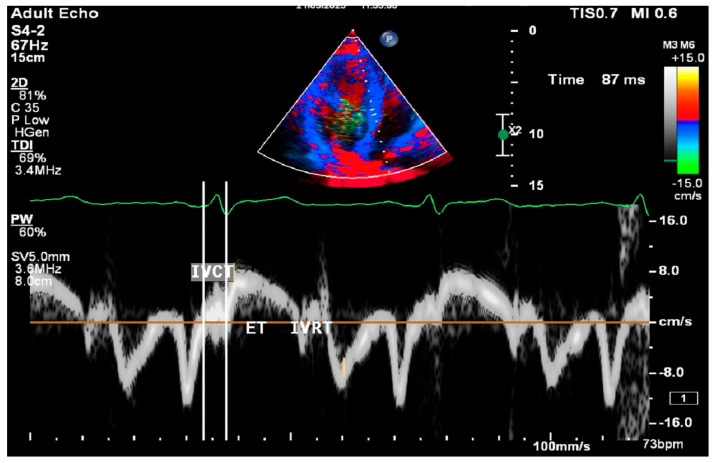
Test image in conventional M-mode, B-mode, and Doppler parameters.
